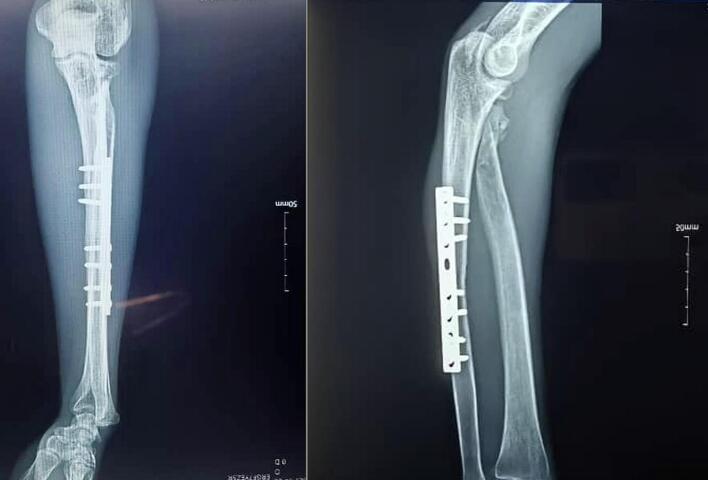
Postoperative radiographs at final follow-up: fracture healing achieved. Major heterotopic ossifications. The radial head is healed and encapsulated within the intact annular ligament.
